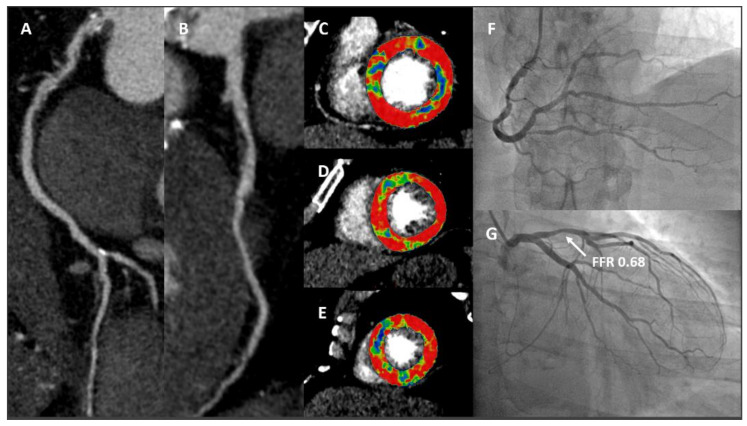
A 61-year-old male smoker with hypertension and dyslipidaemia recently developed exertional chest pain. A cCTA showed severe stenosis at the proximal RCA (Panel (A)) and moderate stenosis (60–70% of lumen area reduction) at the proximal LAD (Panel (B)). Panels (C–E): stress CTP acquired with dynamic acquisition revealed hypoperfused segments after adenosine injection at the mid to apical anterior and anteroseptal walls, basal inferolateral wall and mid to apical inferior and inferoseptal walls. Panels (F,G): ICA showing severe stenosis at the proximal RCA (F) and moderate stenosis at the LAD (G) with abnormal invasive FFR values. *cCTA: cardiac computed tomography angiography; RCA: right coronary artery; LAD: left anterior descending; CTP: computed tomography perfusion; ICA: invasive coronary angiography; FFR: fractional flow reserve.*
